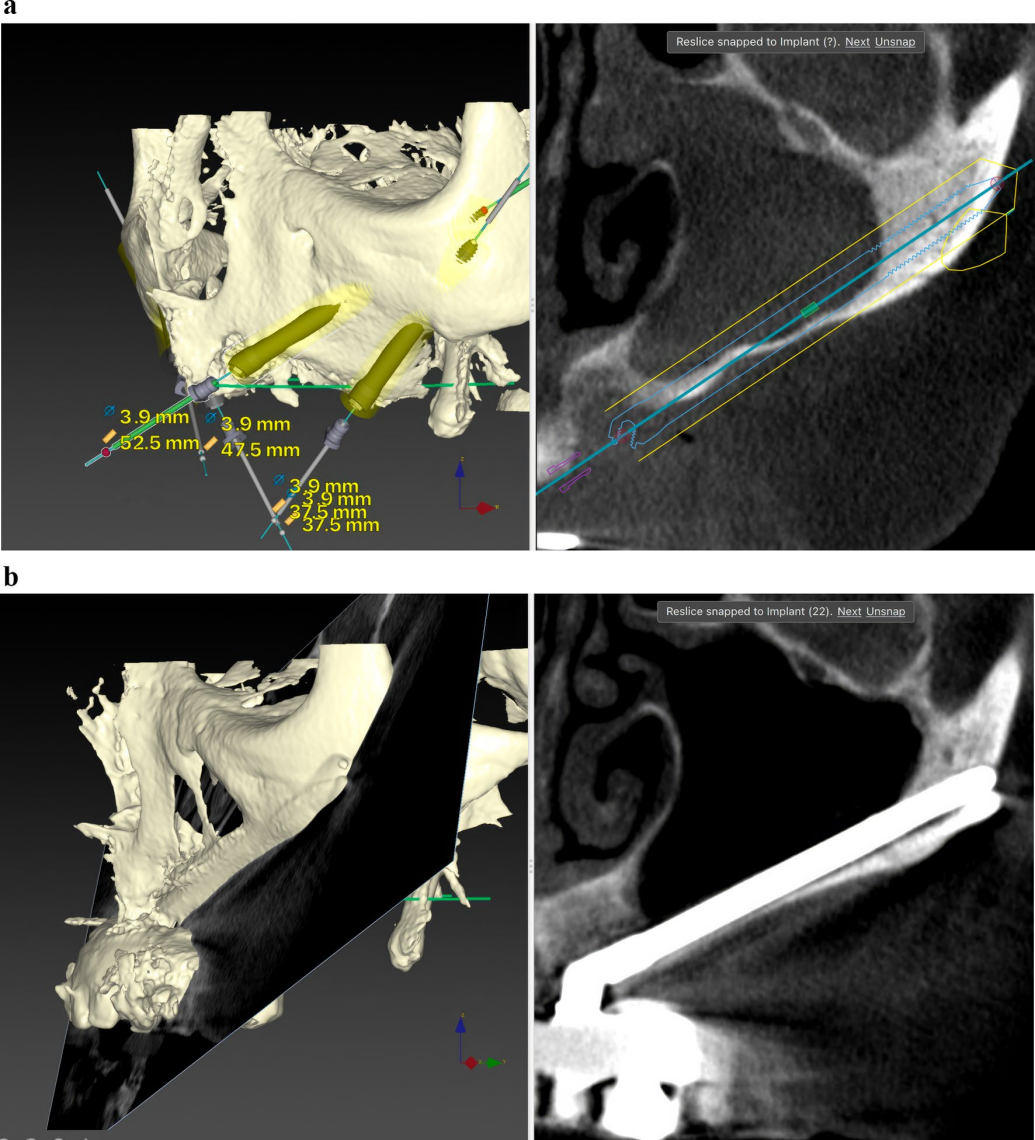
a This heavy smoker patient (P6) presented, as a consequence of implant failure and previous augmentation surgeries, multiple bony discontinuities in the sinus and nasal floor on radiological examination. As observed in the oblique CBCT slice taken in the direction of the left anterior implant, both the maxillary sinuses and the ostium are opaque. The patient had no clinical symptoms. b The same patient (P6) in a presented this CBCT image 18 months after surgery performed according to the recommendations of the ZAGA protocol and using a Straumann ZAGA Round implant. The authors need to determine the reasons for the improved patency at both the sinuses and the ostium. Note also the spontaneous healing of the initial osseous defect at the level of the left incisors
